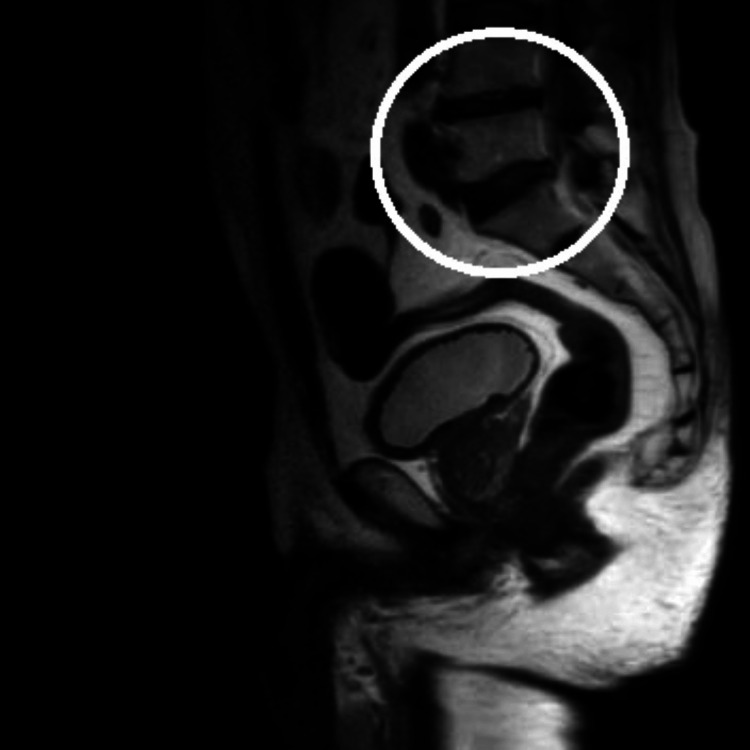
MRI lumbar spine MRI: Magnetic resonance imaging The circle denotes reduced disc height at the L4-L5 level and disc desiccation with diffuse disc bulges noted at the L4-L5 level.
